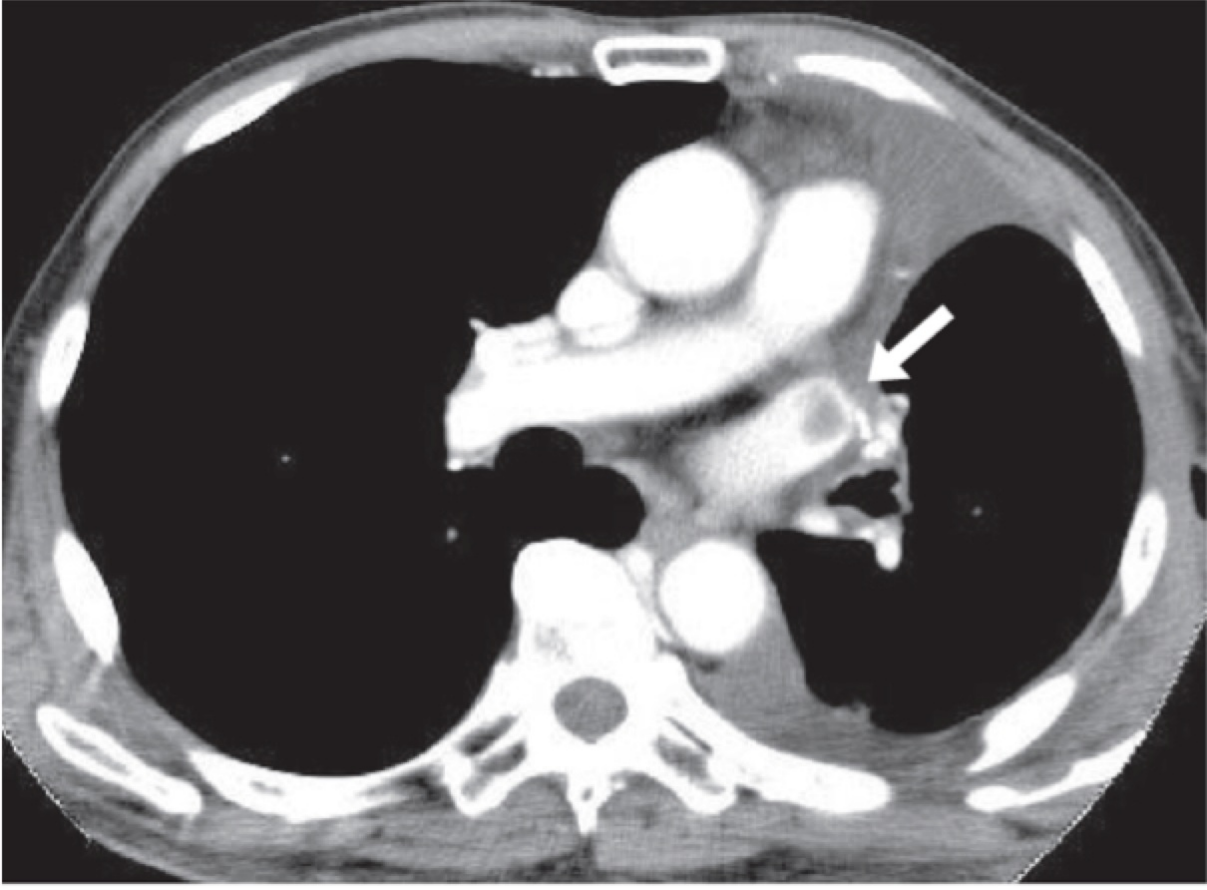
CT scan of the chest with IV contrast A thrombus (white arrow) in the left superior pulmonary vein stump after left upper lobectomy [6].
